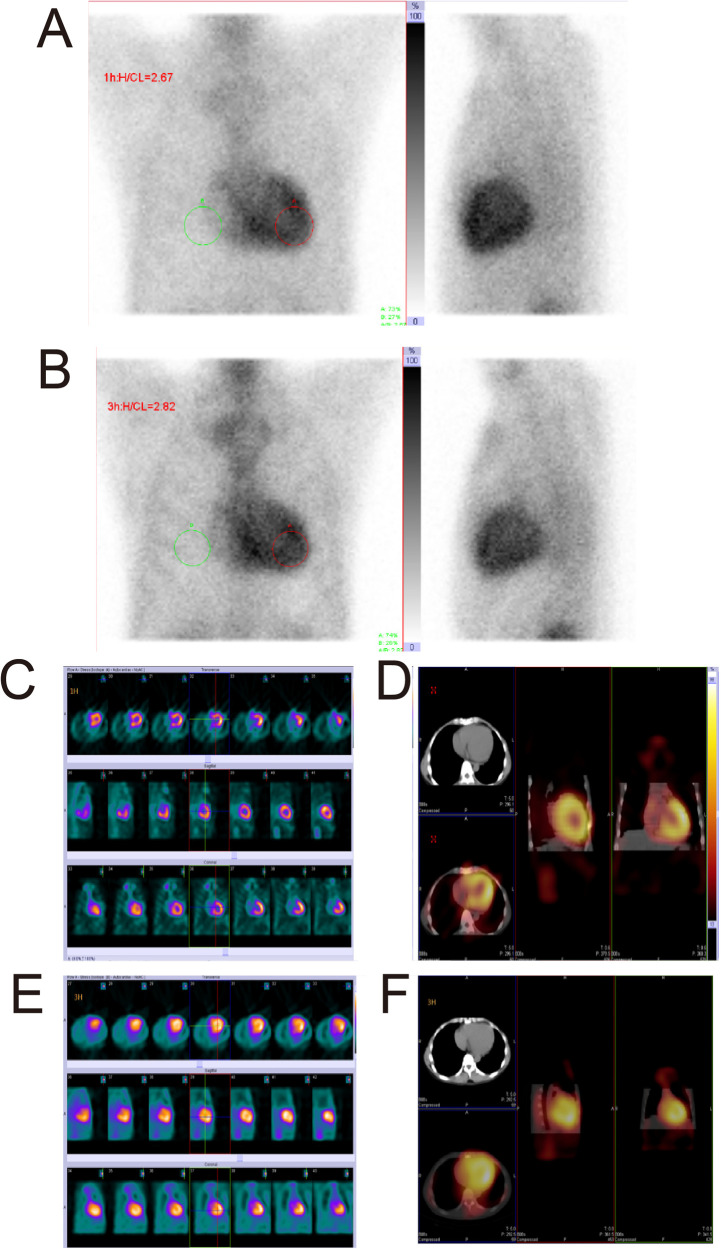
99mTc-PYP myocardial scintigraphy and SPECT/CT fusion at 1 and 3 h demonstrating progressive myocardial uptake. (A) Planar ^99mTc-PYP myocardial scintigraphy 1 h after tracer injection (anterior and lateral views) shows mild uptake in the cardiac region, with only weak radiopharmaceutical accumulation. (B) At 3 h postinjection, planar anterior and lateral views demonstrate substantial cardiac uptake, with prominent radiopharmaceutical accumulation in the anterior wall. The Perugini visual score is grade 3, indicating a high likelihood of transthyretin amyloid cardiomyopathy (ATTR). (C) Transverse, sagittal, and coronal SPECT images at 1 h show mild but definite radiotracer uptake in the cardiac region, suggesting early myocardial amyloid deposition. (D) SPECT/CT fusion images at 1 h confirm that the radiotracer uptake is localized to the myocardium, supporting the presence of amyloid deposits. (E) Transverse, sagittal, and coronal SPECT images at 3 h reveal markedly increased radiopharmaceutical uptake throughout the cardiac region, with more pronounced accumulation in the myocardial tissue, indicative of more advanced myocardial amyloidosis. (F) SPECT/CT fusion images at 3 h highlight intense myocardial uptake of the radiotracer, a pattern consistent with transthyretin amyloid cardiomyopathy.
